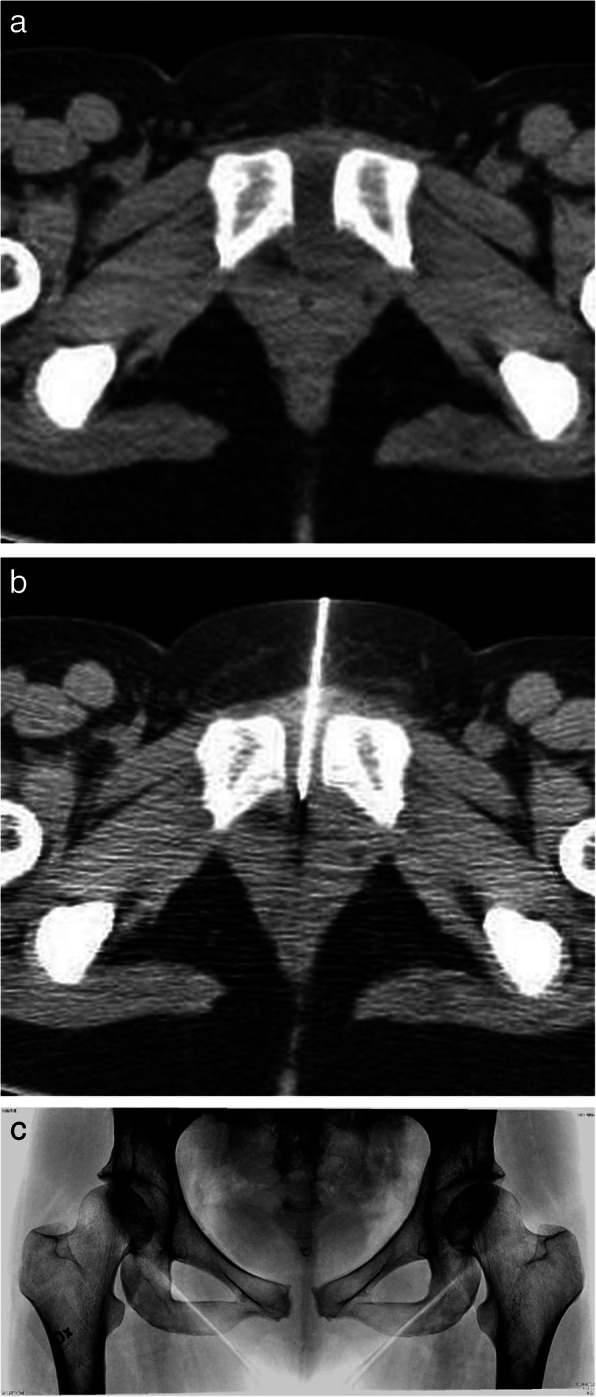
a A 38-year-old year woman. First and normal delivery of a foetus weighing 4530 g. Post delivery onset of pains around the symphysis, malaise and inability to move and walk. High fever and CRP 270. CT scan showed an abscess of the symphysis 4 × 2 × 5 cm. *CRP*, c-reactive protein, *CT*, Computer Tomography. b The symphysis was aspirated under ultra-sound guidance acutely. A new aspiration of 6 ml pus, was done under CT scan guidance five days later. Both cultures revealed Staph. Aureus. Treatment was initiated from day two. *CT*, Computer Tomography. c Six months post-partum, the patient had still symphyseal pains on walking. Radiogram shows a widened symphysis
